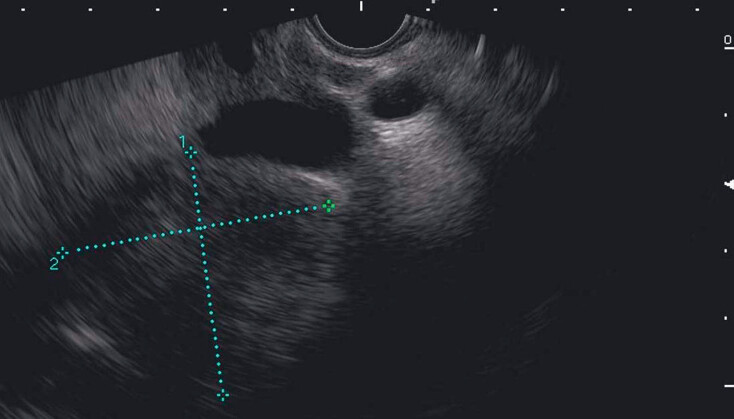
Endoscopic ultrasound findings were consistent with those of CT.
